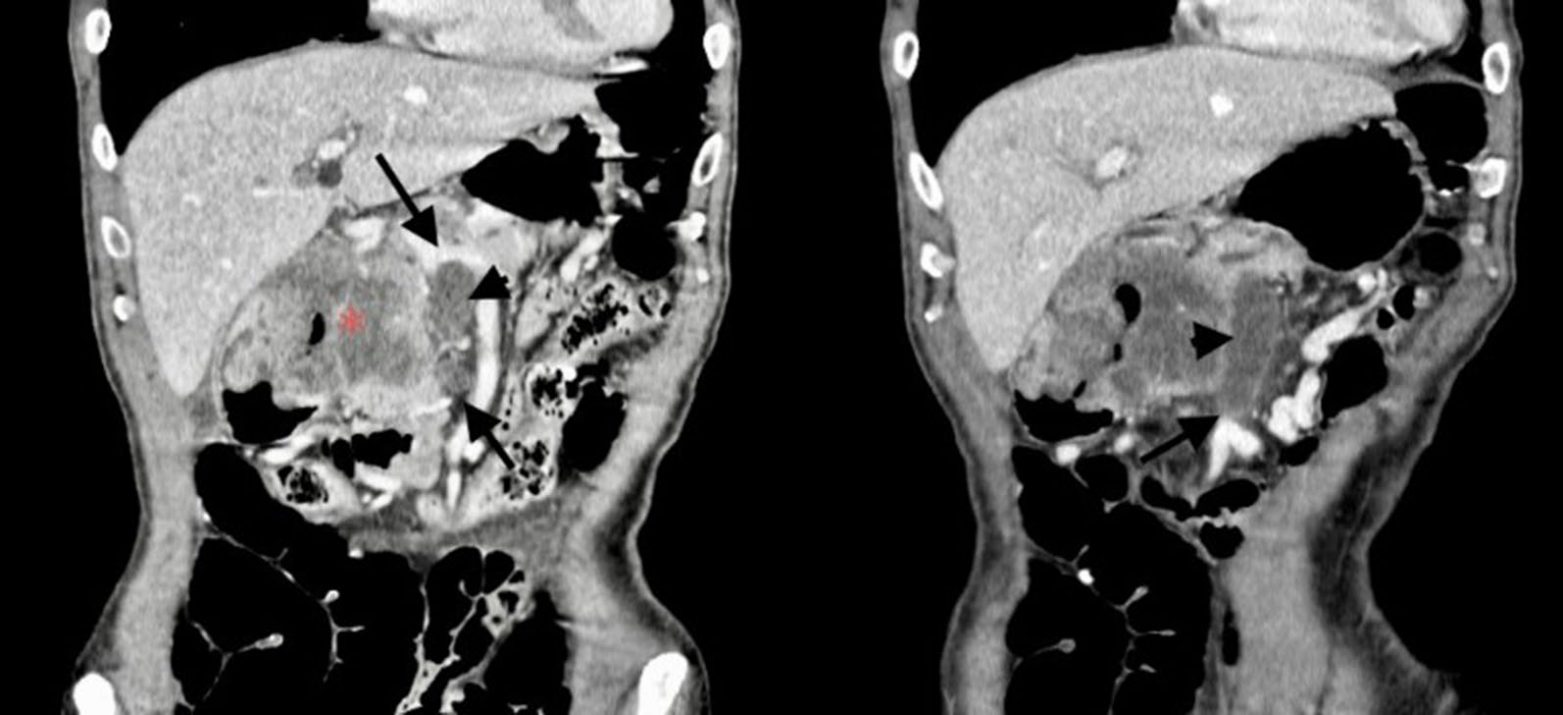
Abdominal enhanced CT. Approximately 10 cm tumor with poor contrast effect was observed in the pancreatic head area (*). A tumor thrombus (arrowhead) of about 6 cm was observed from the superior mesenteric vein (up arrow) to the junction of the portal splenic vein (down arrow)
